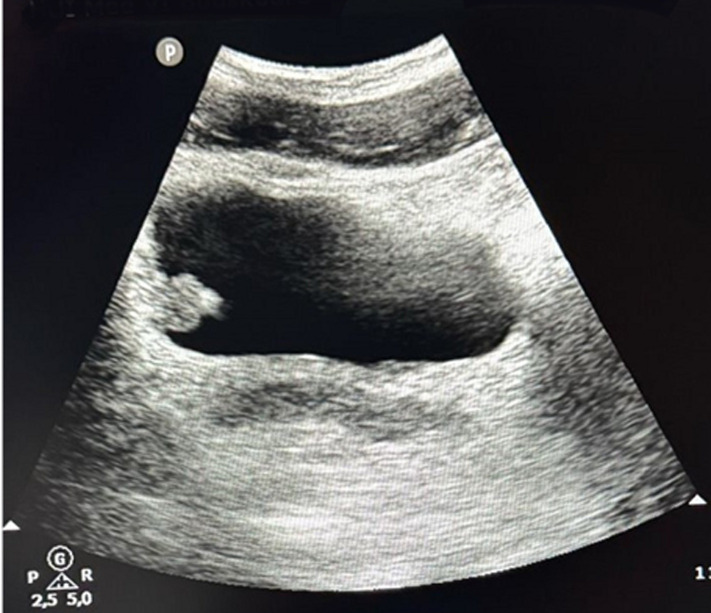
a bladder ultrasound revealed a polypoid mass arising from the right lateral wall
